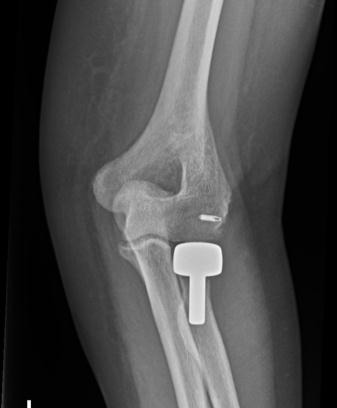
Anteroposterior and lateral radiographs after radial head replacement and lateral collateral ligament repair.
